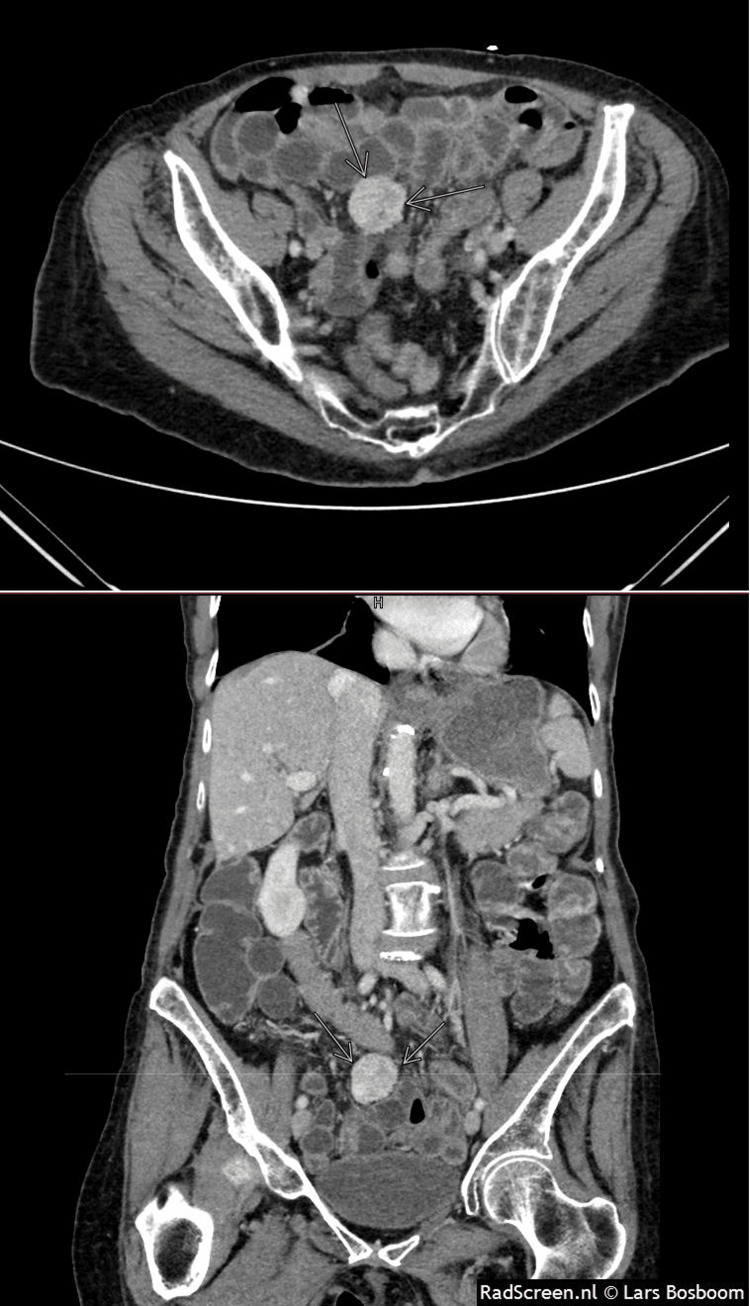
CECT images of the abdomen Axial (top) and coronal (bottom) intravenous (IV) contrast-enhanced computed tomography (CECT) scan images showing an enhancing mesenteric mass.
